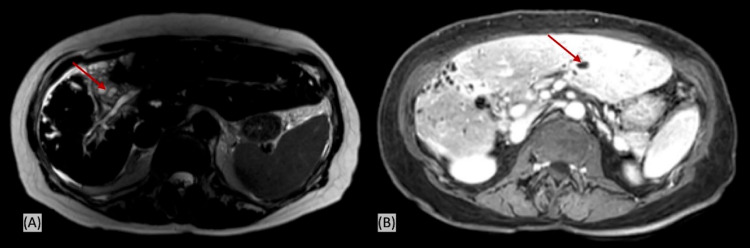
Magnetic resonance cholangiopancreatography (MRCP) showing diffuse common bile duct stricturing and biliary duct dilatation Panel A: T2-weighed image; panel B: post-contrast T1-weighted image with fat saturation
